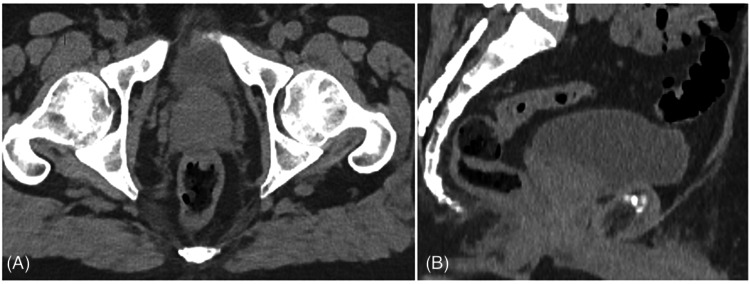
(A) Axial and (B) sagittal CT images confirming the absence of foreign bodies or high-density material.
